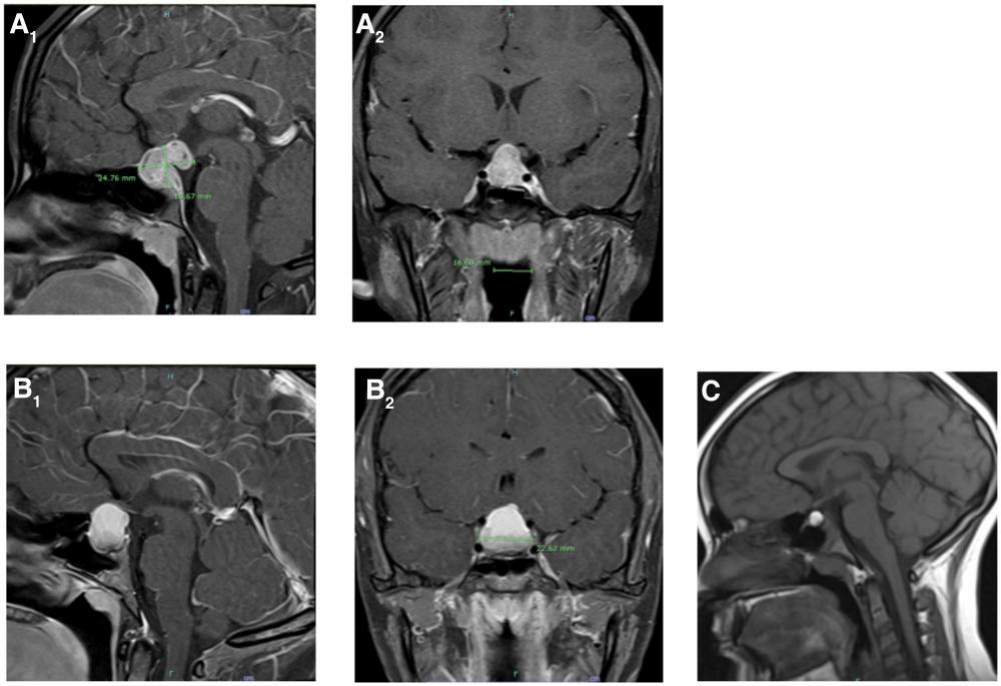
Preoperative imaging studies. (A) Patient 1 MRI sagittal and coronal views at diagnosis showing a 1.7 × 2.5 × 1.9 cm heterogeneously enhancing mass involving the sella and the suprasellar regions. It is isointense on the T1-weighted images and hypointense on the T2-weighted images with remodeling of the dorsum sella and absent posterior pituitary bright spot. (B) Patient 2 MRI sagittal and coronal views at diagnosis showing a 1.6 × 2.2 × 2.3 cm heterogeneously T1 hyperintense lesion expanding the sella with suprasellar expansion, spuriously deviated infundibulum, and splaying of the prechiasmatic optic nerves. (C) Patient 2 noncontrast MRI 5 years prior to diagnosis showing 1.4 cm Rathke cleft cyst. Abbreviation: MRI, magnetic resonance imaging.
